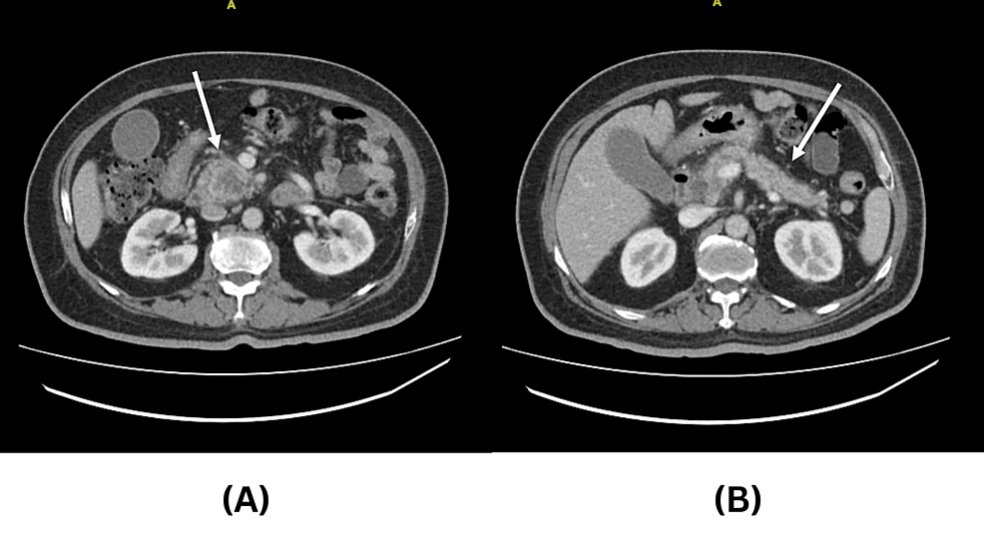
Axial CT scan for patient 1 (A) Axial CT showing the head/uncinate process of the pancreas with the tumor (arrow), (B) axial CT scan showing the body and tail of the pancreas clear of any lesion.
